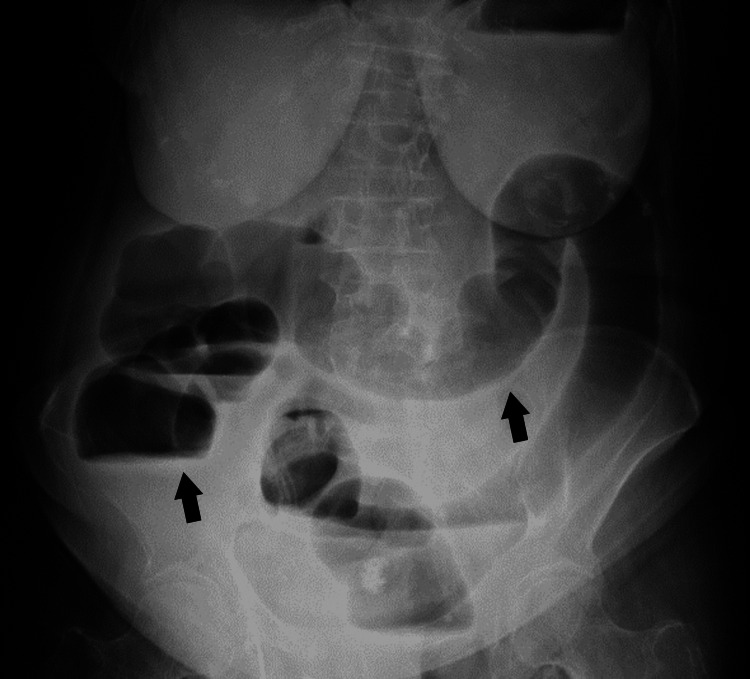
X-ray image of the abdomen X-ray image of the abdomen showing marked dilatation of the entire colon with air-fluid levels (arrows).
